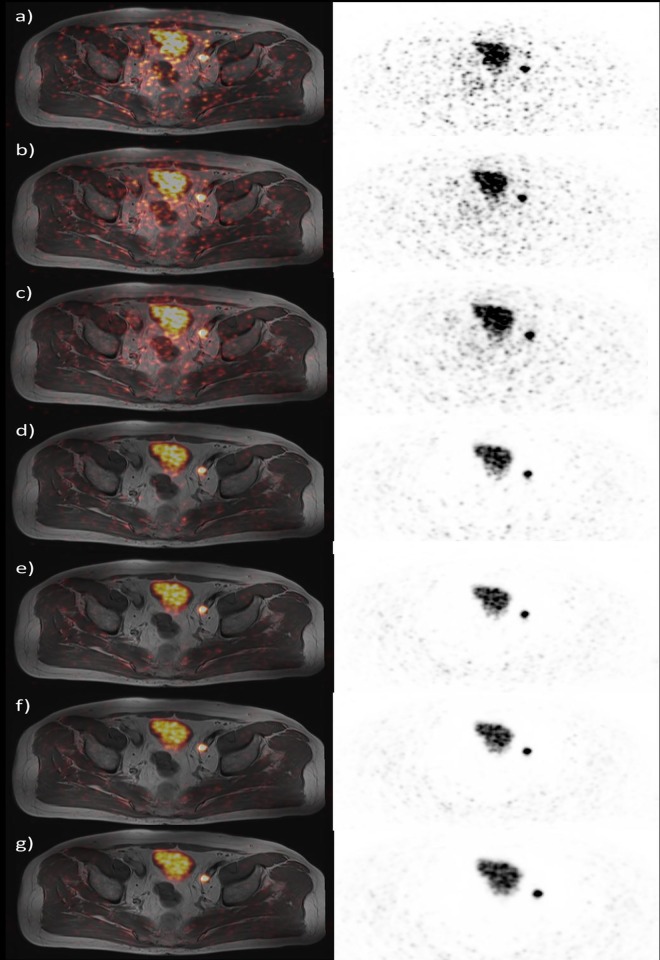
Image quality of ^68^Ga-HBED-CC-PSMA PET/MRI of a patient with recurrent PCa in an ilical lymph node. Images were acquired at 3 h p.i.. Left panel: PET/MR fusion image, right panel: PET image of the PET/MR hybrid imaging system. Acquisition times were a) 1 min, b) 2 min, c) 3 min, d) 4 min, e) 6 min, f) 8 min, and g) 10 min. PET image quality rises with increasing acquisition times and reaches a plateau at an acquisition time of 4 min. The halo artifact first occurs at image acquisition times of 4 min.
